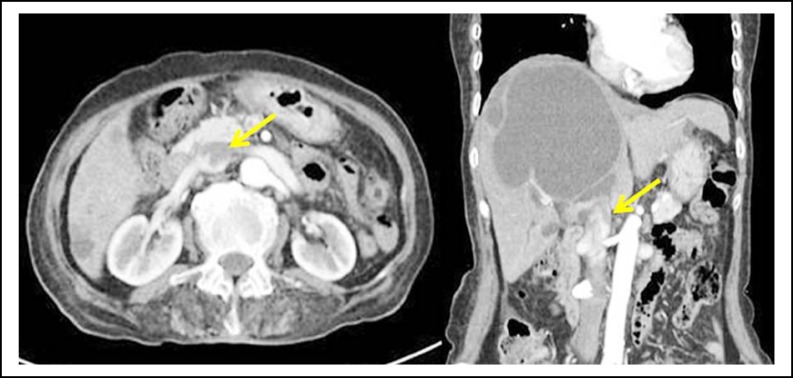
Computed tomography scan revealing multiple variable-sized cysts in both the kidneys and liver. The hepatic level of the IVC is compressed by massive liver cysts. IVC thrombosis is observed
